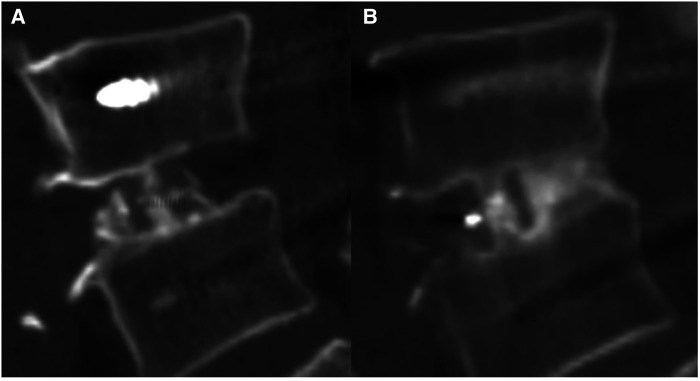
A 71-year-old Male patient received cage implantation after surgery for lumbar spinal stenosis. (A) Sagittal lumbar CT scan performed at postoperative 3 days revealed good bone filling in the inner cage zone but no bone graft in the posterior cage zone. (B) CT at postoperative 3 years showed bone remodelling and passage of the bone trabecula in the inner cage zone and the posterior cage zone.
